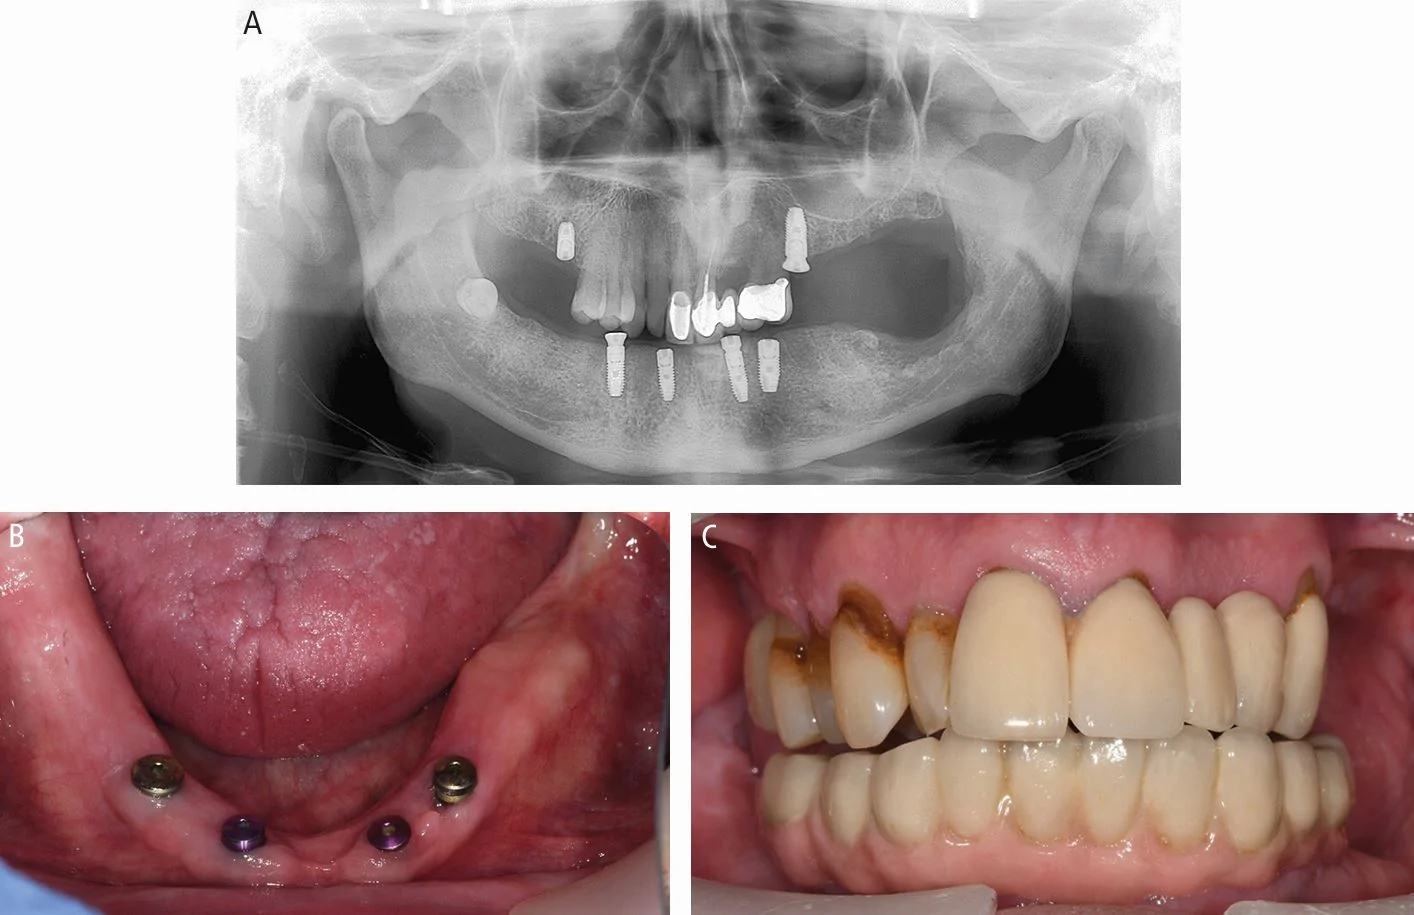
Successful rehabilitation of area with implant-retained fixed bridges. (A) Panoramic radiograph of osteoradionecrosis lesion healing three years post debridement and following implant placement. (B) Clinical photograph of mandibular implants with defect from healed ORN lesion. (C) Clinical photograph of implant retained fixed bridges *in situ*
